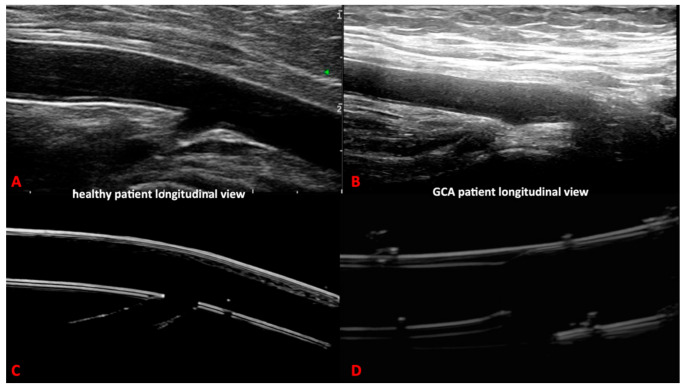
Comparison between ultrasound images of the axillary artery of the 3D printed models (C,D) and real ultrasound images in healthy and giant cell arteritis patients (A,B).
